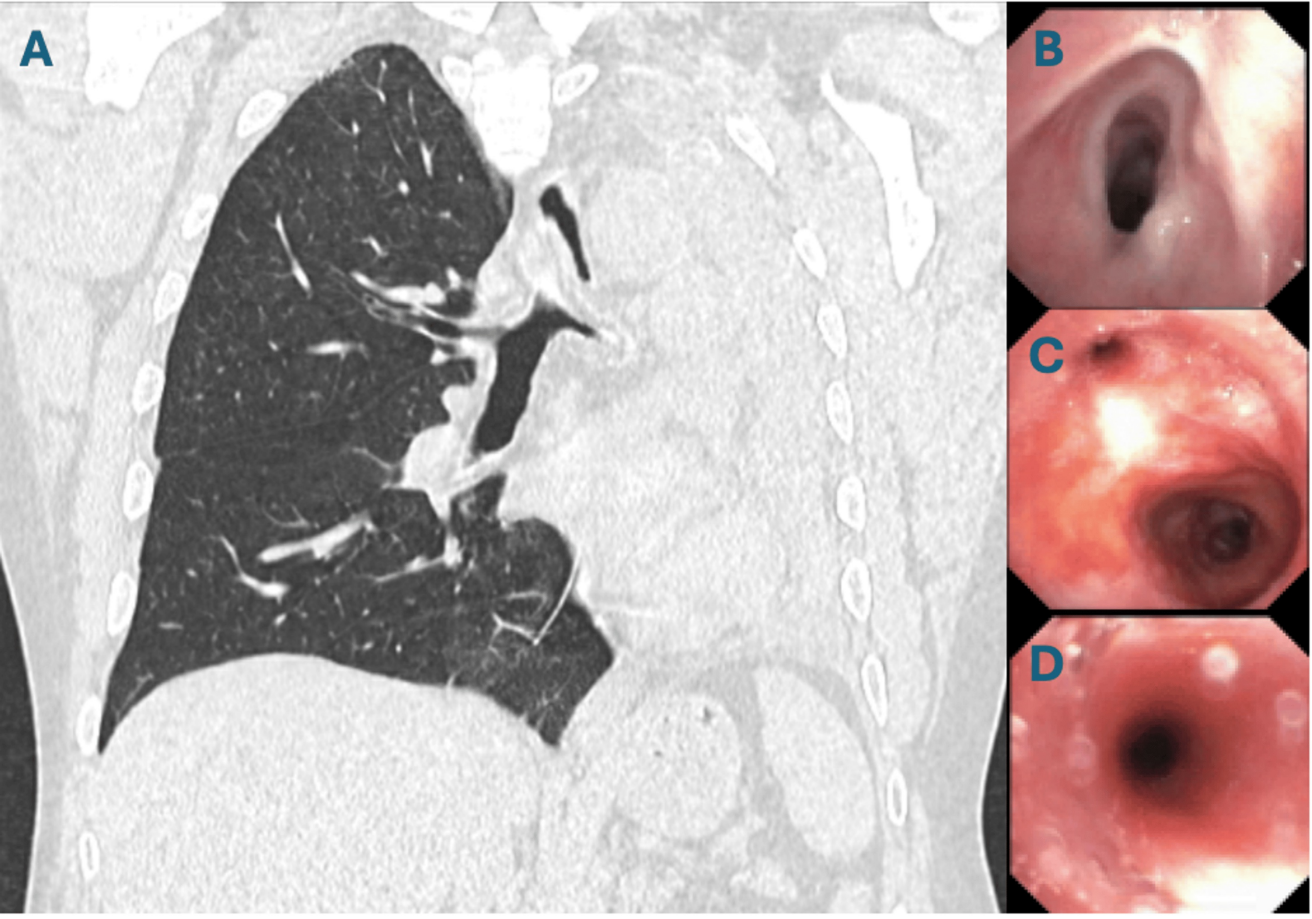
Imaging and bronchoscopic evidence of airway stenosis in mucous membrane pemphigoid (A) Coronal chest CT demonstrating complete collapse of the left lung with ipsilateral volume loss and mediastinal shift, caused by severe narrowing of the left main bronchus. (B) Bronchoscopic view of high-grade tracheal stenosis with circumferential mucosal thickening. (C-D) Progressive narrowing of the left main bronchus with friable, inflamed mucosa and near-complete luminal obstruction, consistent with advanced airway-predominant MMP.
